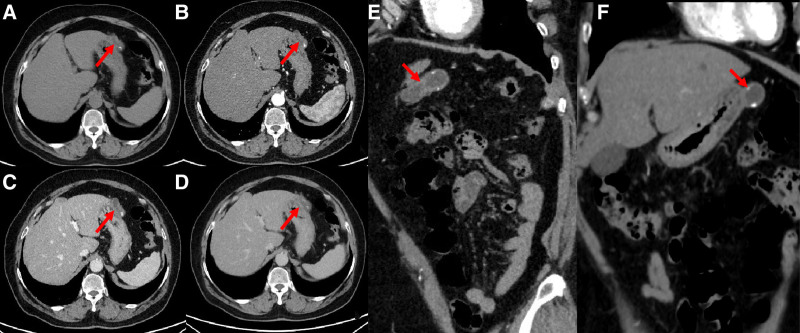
(A–D) represented axial CT plain scan, arterial phase, venous phase and delayed phase of enhancement scan, (E and F) represented oblique sagittal and coronal arterial enhancement, respectively, showing another cystic lesion protruding from gastric corpus of greater curvature, with a larger axial cross-sectional area of 36 mm × 17 mm. Punctate calcifications were found in the cyst wall. The CT features of cyst wall and cyst content were similar to the lesion of gastric antrum.
